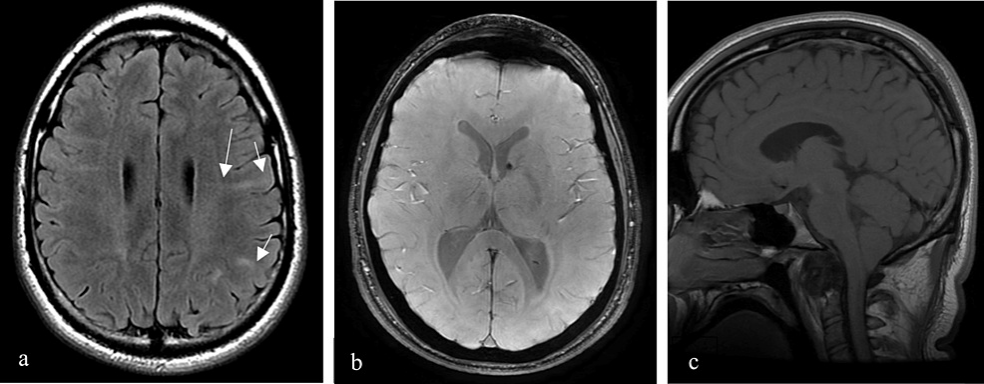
Brain MRI with axial T2FLAIR-weighted image (a), axial T2*-WI (b) and sagittal T1-WI (c). Cortical and subcortical tubers (short arrow) and radial migrating bands (long arrow) in both cerebral hemispheres are shown on axial T2FLAIR-WI (a). A small calcified subependymal hamartoma in the frontal horn of the left lateral ventricle is better depicted on axial T2*-WI (b). Sagittal T1-WI (c) shows a Chiari I malformation, with a short clivus and caudal descent of cerebellar tonsils, compressing the posterior surface of the medulla. It also reveals a space-occupying lesion at the atlanto-axial joint.
